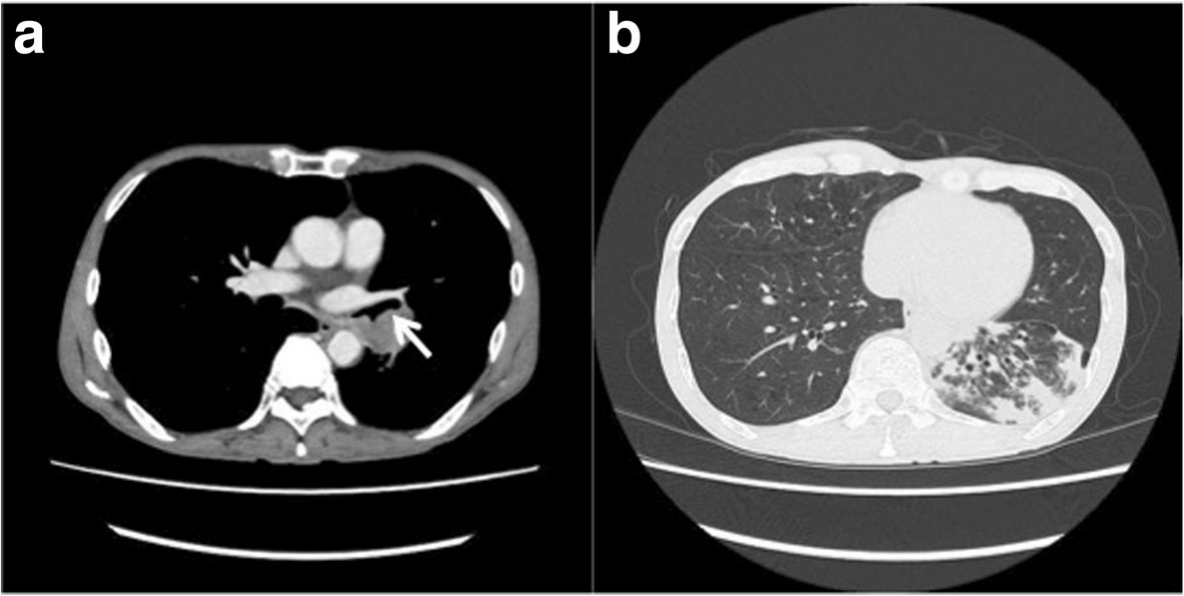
Computed tomography (CT) scan showed solid tumor of 40 mm in diameter in left lower bronchus which involved lingular division bronchus (solid arrow) (a), which also obstructed the lower bronchus and caused obstructive pneumonia of left lower lobe (b)
